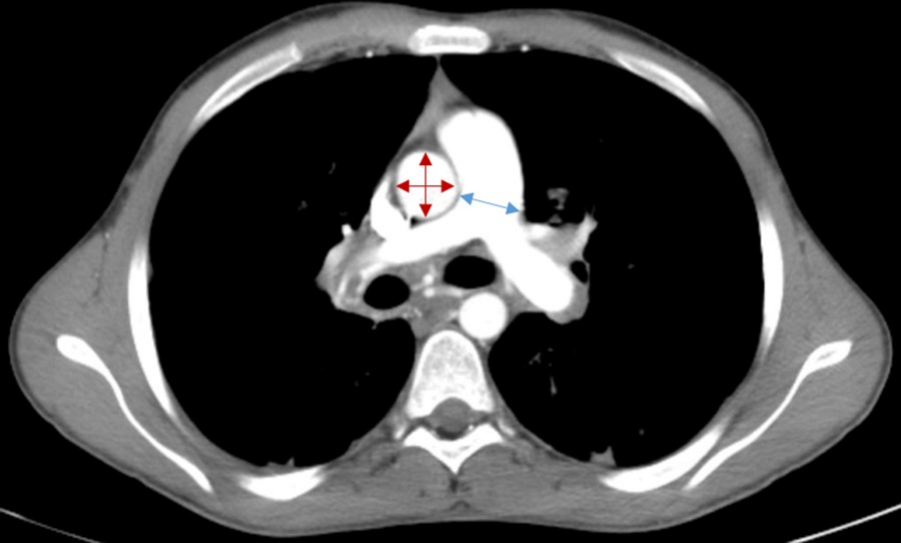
Pulmonary arterial enlargement in severe cystic fibrosis. A representative axial image of a CT scan at the level of the pulmonary artery (PA) bifurcation. The diameter of the PA is measured at the location indicated by the blue arrow. The diameter of the ascending aorta (A) is calculated using two perpendicular measurements indicated by the red arrows. PA enlargement (PA:A>1) is present in this image.
